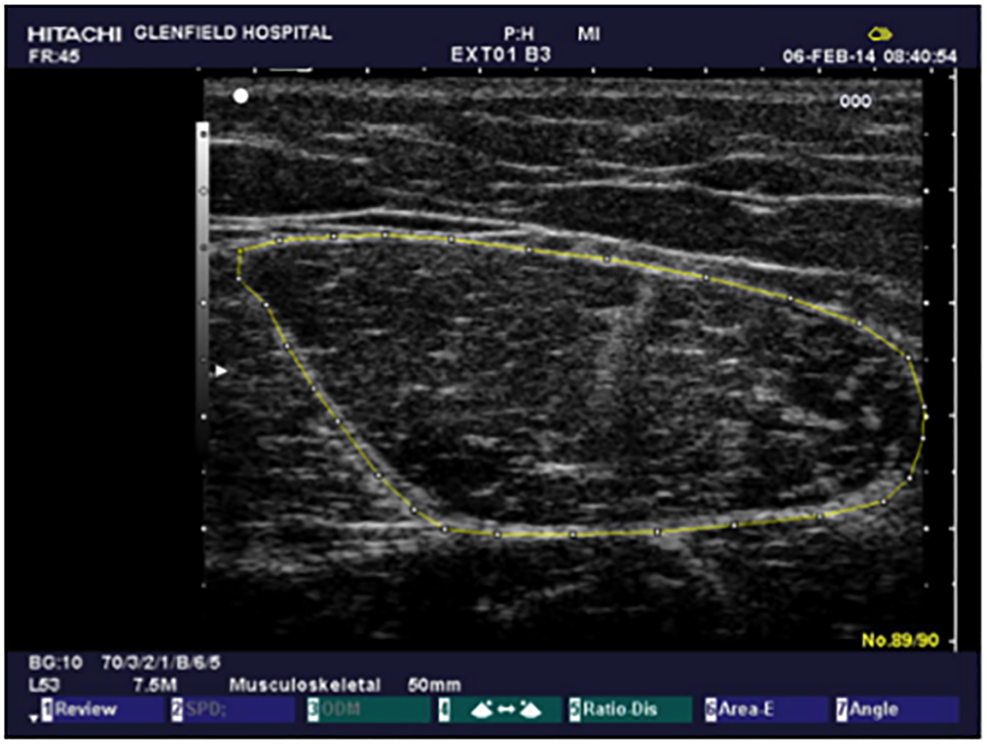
Representative image of rectus femoris cross‐sectional area measured by 2D B‐mode ultrasound.
